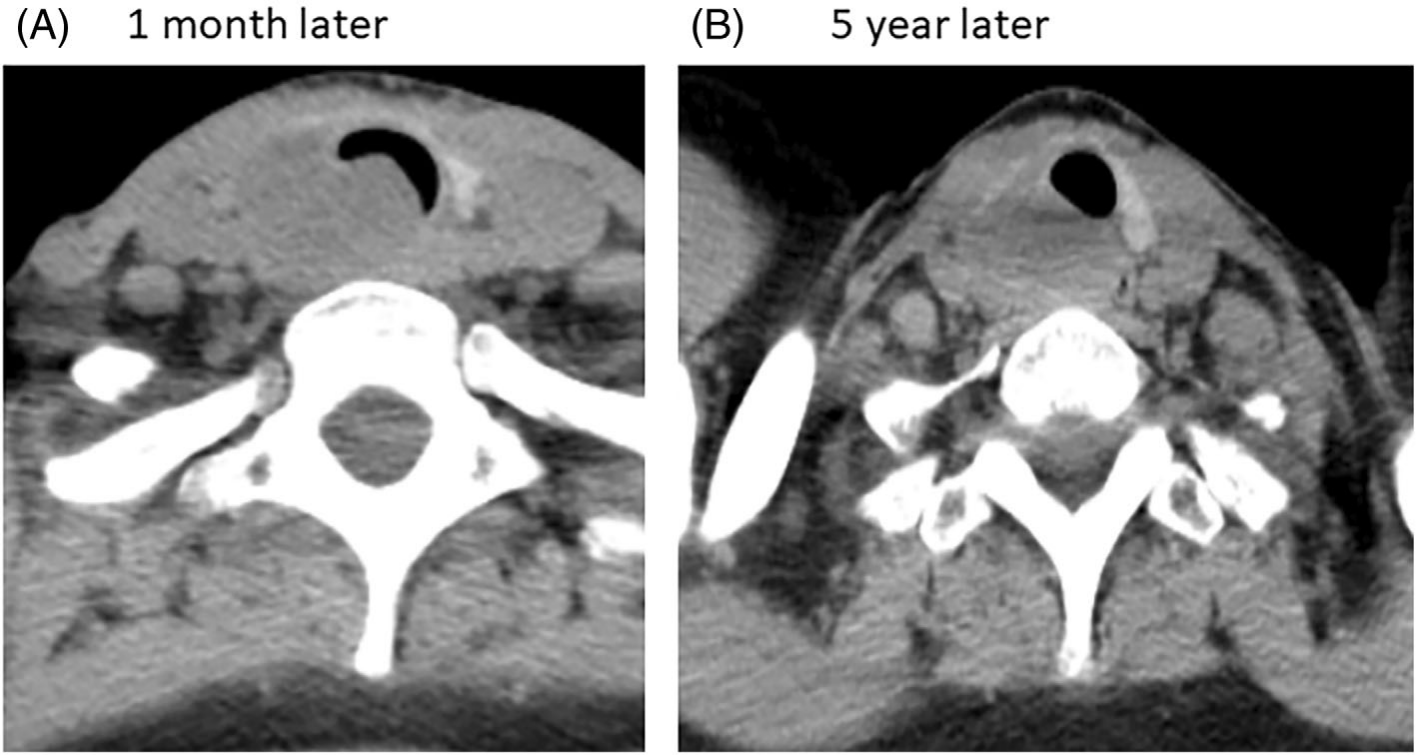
(A) Tumour regrowth in the bronchial lumen was observed 1 month later on relieving intratracheal stenosis. (B) No tendency for tumour growth was observed on computed tomography 5 years later.
